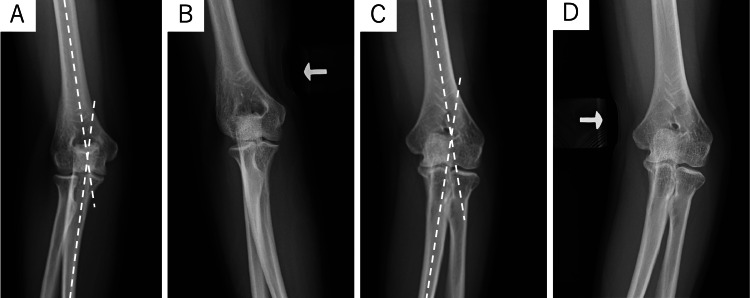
Preoperative radiographs of case one (A) Right AP radiograph. The CA was 12 degrees in the right upper extremity. (B) The right elbow showed no instability during varus stress testing. (C) Left AP radiographs showed an 18-degree cubitus varus deformity. (D) Left AP radiograph under varus stress. The left elbow showed 8 degrees of varus instability.
